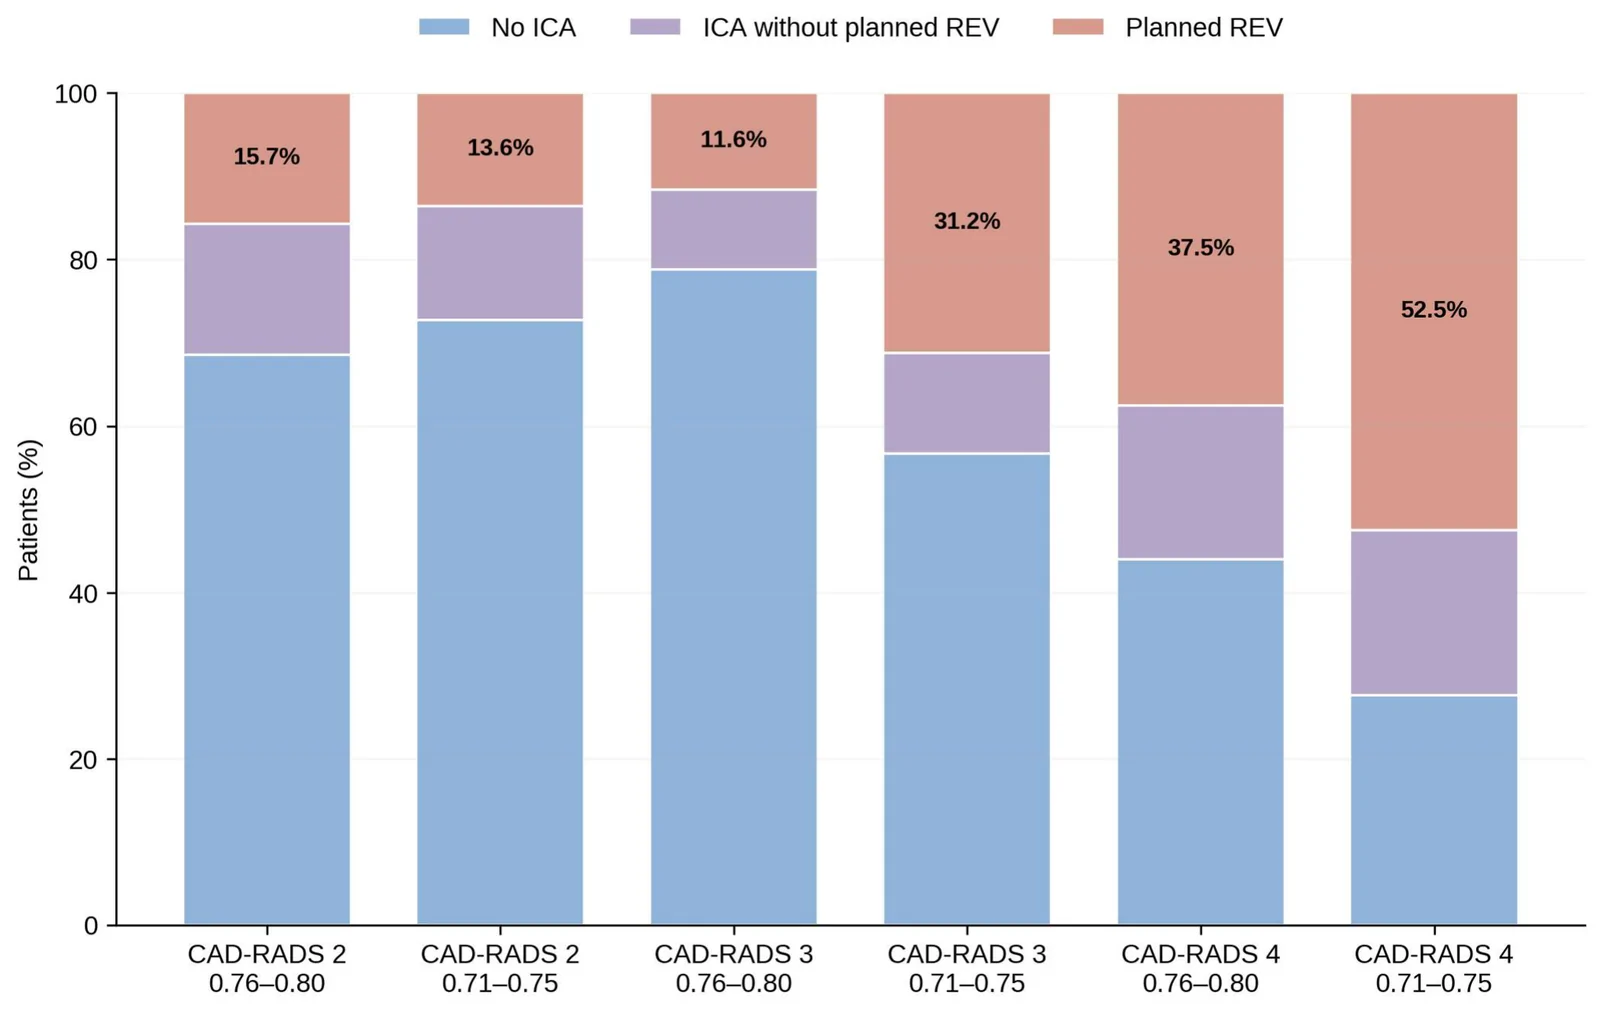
Downstream management pathways across combined CAD-RADS and CT-FFR strata. Bars show the proportions of patients who did not undergo ICA, underwent ICA without planned revascularization, or underwent planned revascularization in each CAD-RADS and CT-FFR stratum. Percentages displayed within the upper segment indicate planned revascularization rates. ICA = invasive coronary angiography; REV = revascularization; CT-FFR = coronary CT angiography–derived fractional flow reserve; CAD-RADS = Coronary Artery Disease Reporting and Data System.
